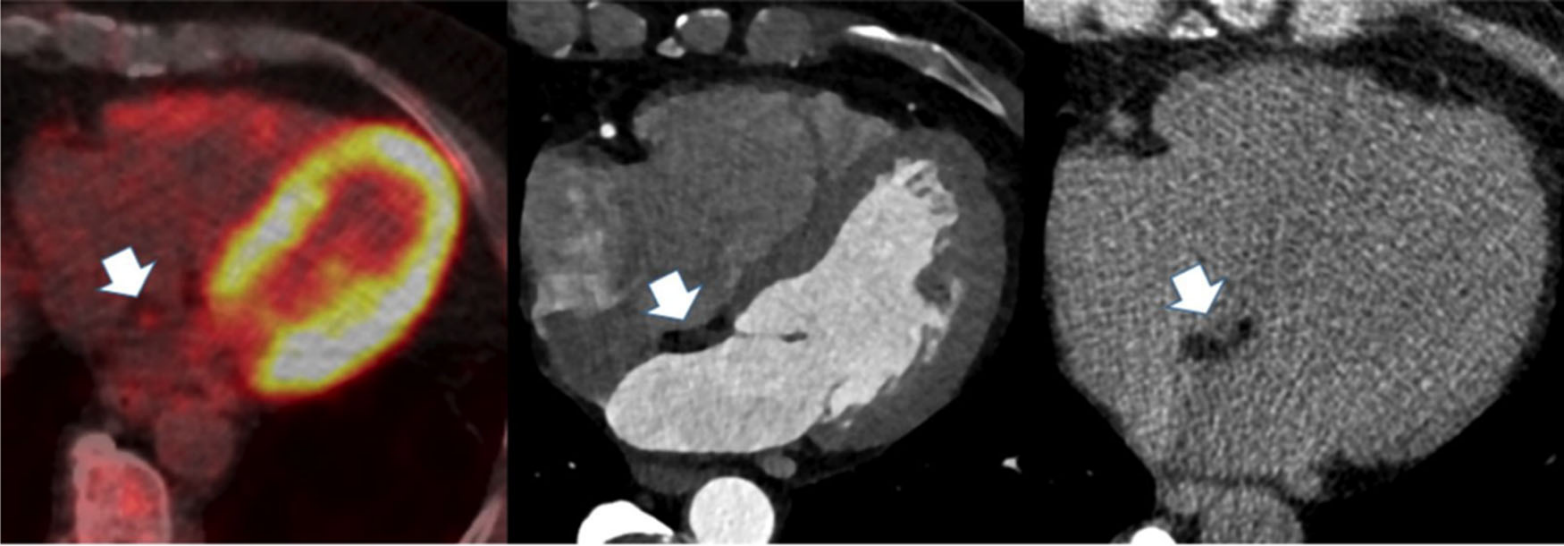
A 64-year-old male (BMI, 24.2 kg/cm^2^) with high LHIS density (mean + 24 HU). Mild [^18^F]FDG tracer uptake (left panel) central. White arrow pointing at LHIS on coronary CTA (mid panel) and unenhanced CAC score (right panel)
